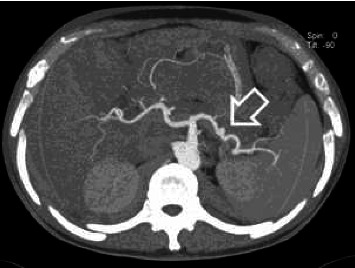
Axial computed tomography angiography of the abdomen shows a saccular pseudoaneurysm of the proximal splenic artery, measuring 6 mm (arrow).
